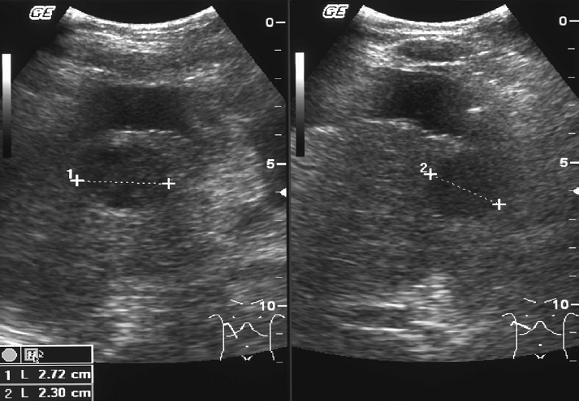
Hypodense lesion (27 × 23 mm) in ultrasound.
